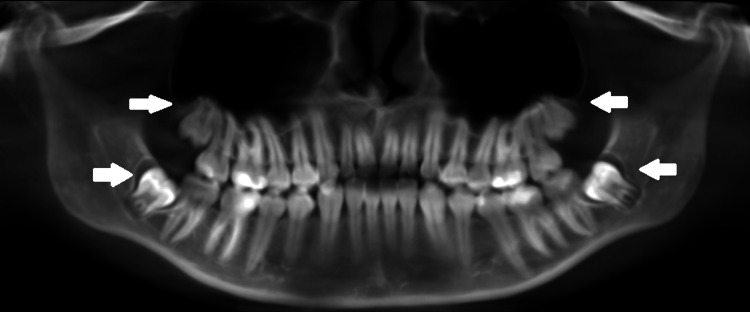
Orthopantomogram generated from the CBCT. White arrows indicating wisdom teeth impaction. CBCT: cone-beam computed tomography
